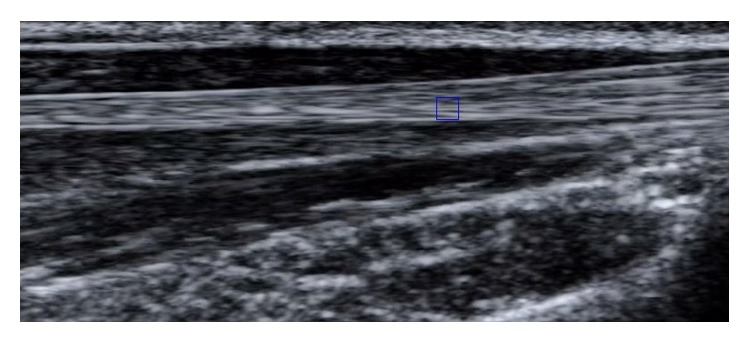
An image of ultrasonic extensor tendon image sequence.
